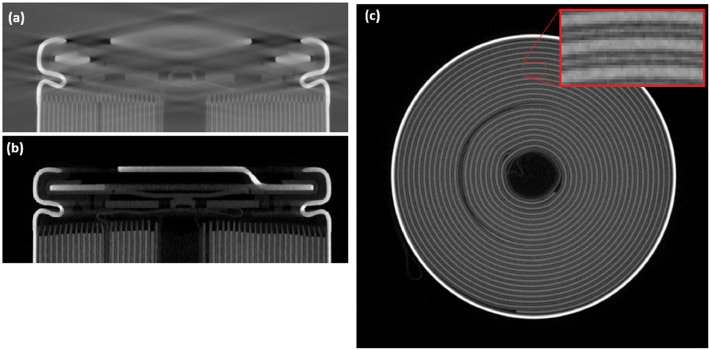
(a) example of poor data quality in a axial view slice due to cone beam artefacts, (b) an example axial view slice without cone beam artefacts, (c) the minimum recommended SNR in the radial view, the in-set image shows electrode layers are separated.
